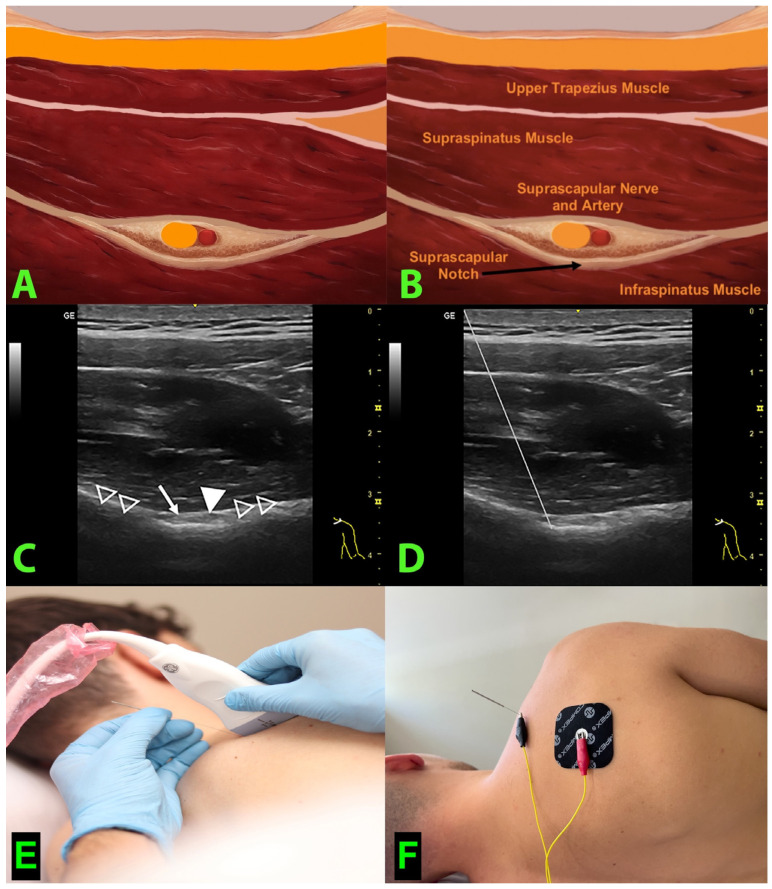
(A) Ultrasound-guided identification and percutaneous electrical nerve stimulation (PENS) of the suprascapular nerve (yellow) located lateral to the suprascapular artery (red) at the suprascapular notch. (B) The upper trapezius muscle is the most superficial, overlying the supraspinatus muscle. The suprascapular notch is located at the deepest part of the suprascapular fossa, where the nerve and artery course adjacent to one another before entering the supraspinous fossa. (C) Ultrasound view of the suprascapular fossa; the bony floor (open arrow) outlines the fossa and guides identification of the suprascapular nerve within the fascial plane. The suprascapular nerve (white arrow) and suprascapular artery/vein complex (arrowhead) can be visualized. (D) Ultrasound view of the needle path (white line) approaching the suprascapular nerve. (E) Ultrasound-guided needle insertion targeting the suprascapular nerve. (F) Application of PENS on the suprascapular nerve with the needle electrode and reference electrode coupled to channel one (yellow) of the ITO ES-160 device.
